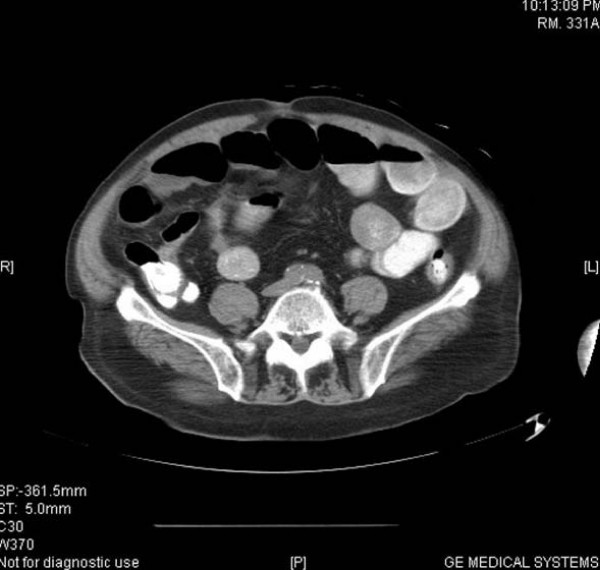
Abdominal CT scan with intravenous contrast showed mild ascites and increased small bowel distention.
